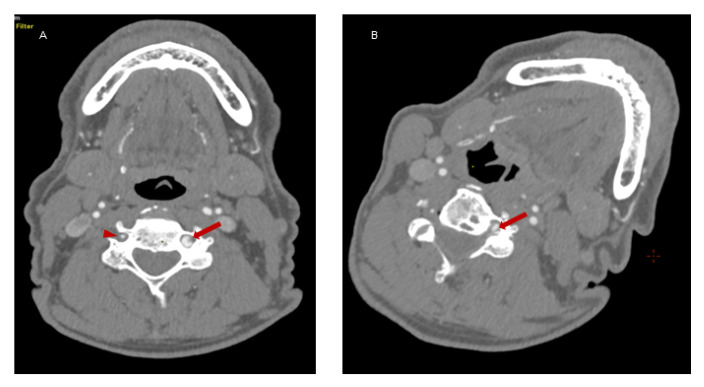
Dynamic CT angiography in the neutral head position and left-side rotation. Panel (A): Axial D-CTA in the neutral head position demonstrates L-VA loop in V2 segment (*red arrow*) at C4 level and R-VA hypoplasia (*red arrowhead*). No L-VA stenosis in this position. Panel (B): Axial D-CTA in left-side head rotation demonstrates the narrowing of L-VA lumen (up to 2 mm × 3.2 mm) that causes a dynamic 60% L-VA stenosis in the loop at C4 level (*red arrow*).
